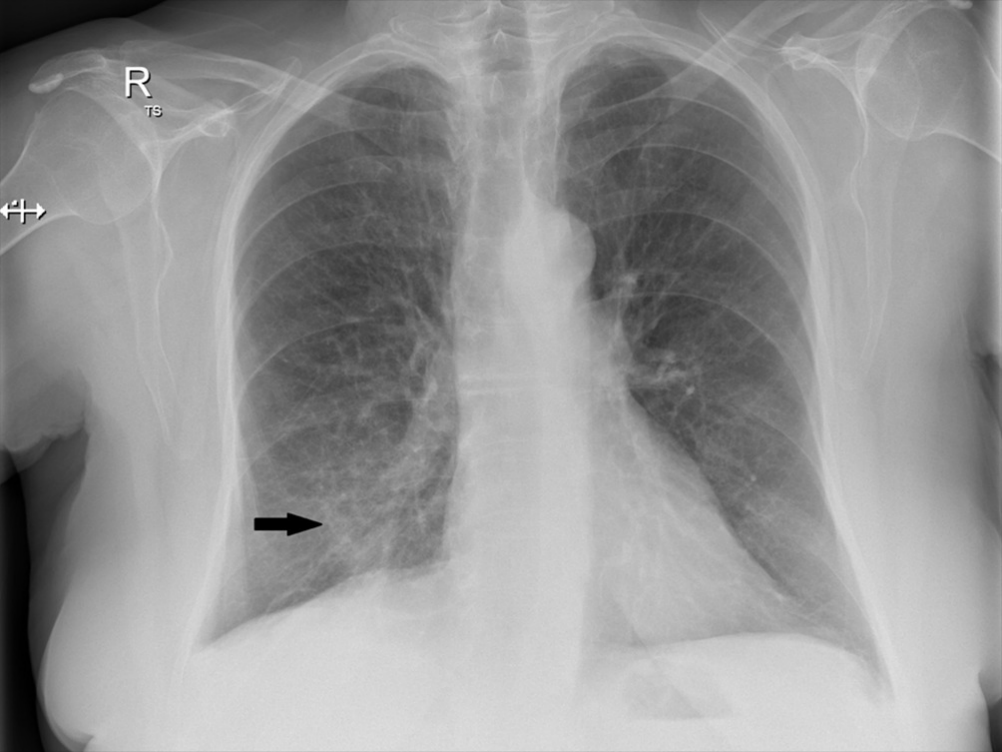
Frontal chest X-ray demonstrating a right lower lobe ill-defined opacity (arrow).
